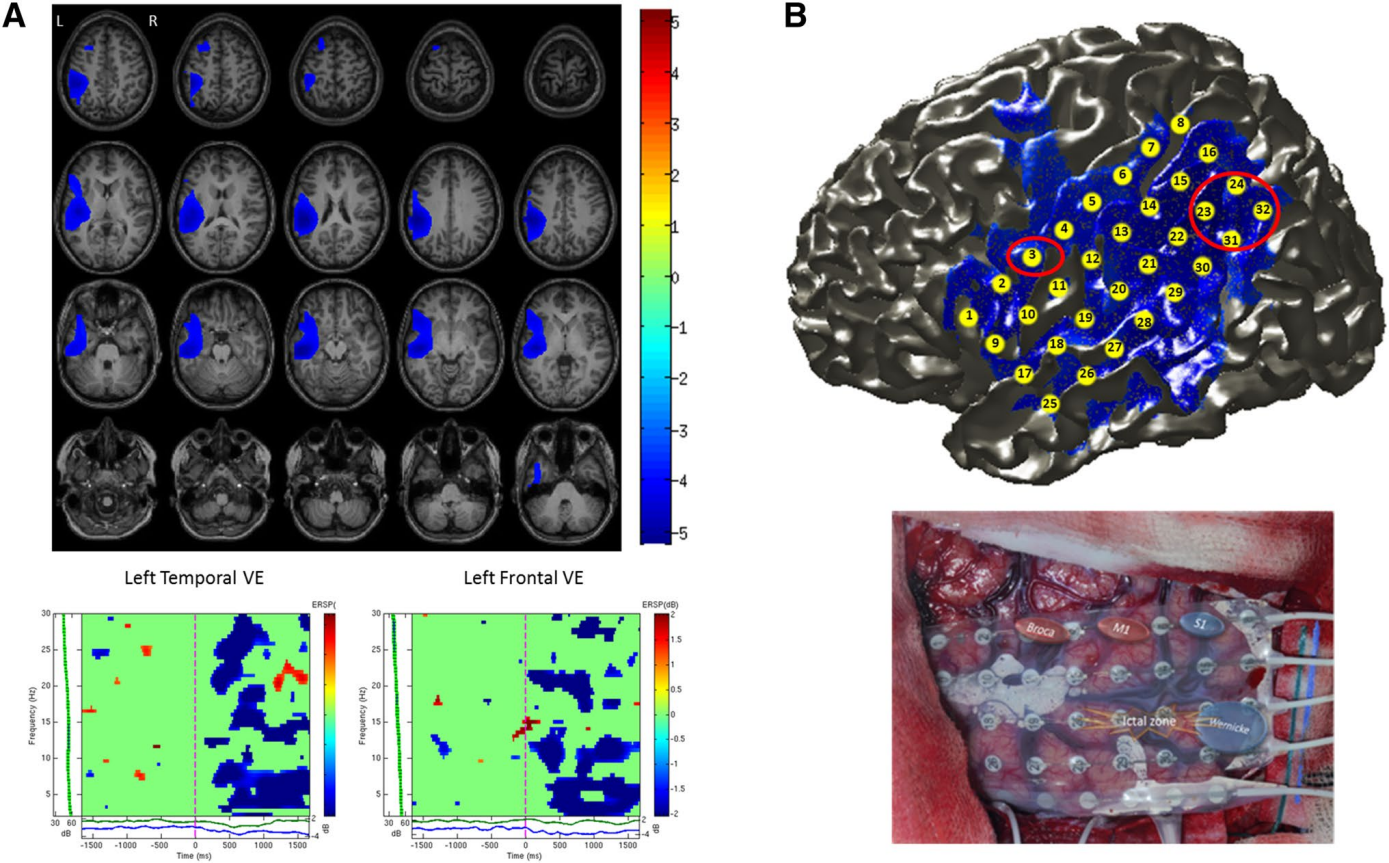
a Beamformer source localization results of verb generation task for an individual patient (Pt. 13) are shown overlaid on axial slices of the patient’s T1 MRI. Sources showing significant decreases in beta oscillatory power (13–30 Hz) from 2.5 s pre- to 2.5 s post-stimulus onset were identified in left temporal and left frontal regions (LI = 64.95). Time–frequency analysis of a virtual electrode (VE) constructed in the left temporal lobe shows an early sustained decrease in beta power during covert verb generation. Similarly, time–frequency analysis of a virtual electrode constructed in the left frontal lobe shows decreases in beta power from around 200 ms during covert verb generation. b Top: Electrode grid co-registered and overlaid on cortical surface of the patients MRI along with MEG beamformer source localization results. Red circles indicate location of Wernicke’s and Broca’s areas as identified during direct cortical stimulation. The peak source of decreased beta power (13–30 Hz) localized by MEG corresponds to Wernicke’s area in this patient. Bottom: Photograph of 32-channel grid shows results from cortical stimulation, where Broca’s area was mapped to contact 3 and Wernicke’s area to contacts 23–24 and 31–32. When these contacts were stimulated the patient had difficulty producing verbs, was confused and stuttered
